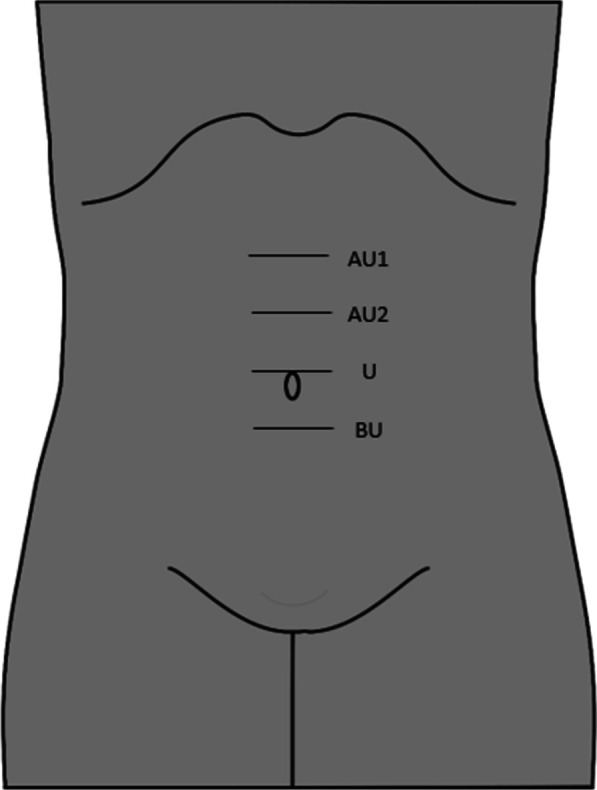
Proposed sites for inter-recti distance measurement using ultrasonographic imaging based on the scoping review results synthesis. AU1: ½ of the superior umbilical border-xiphoid distance; AU2: ¼ of the superior umbilical border-xiphoid distance; U: the superior umbilical border; and BU: ¼ of the superior umbilical border-pubis distance
